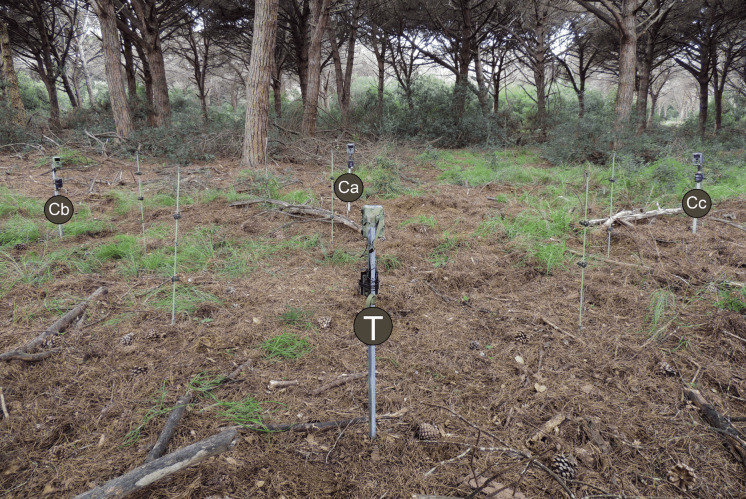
A picture of the trial layout. The overlapped label T indicates the test CT and the labels Ca, Cb, and Cc the three control CTs
